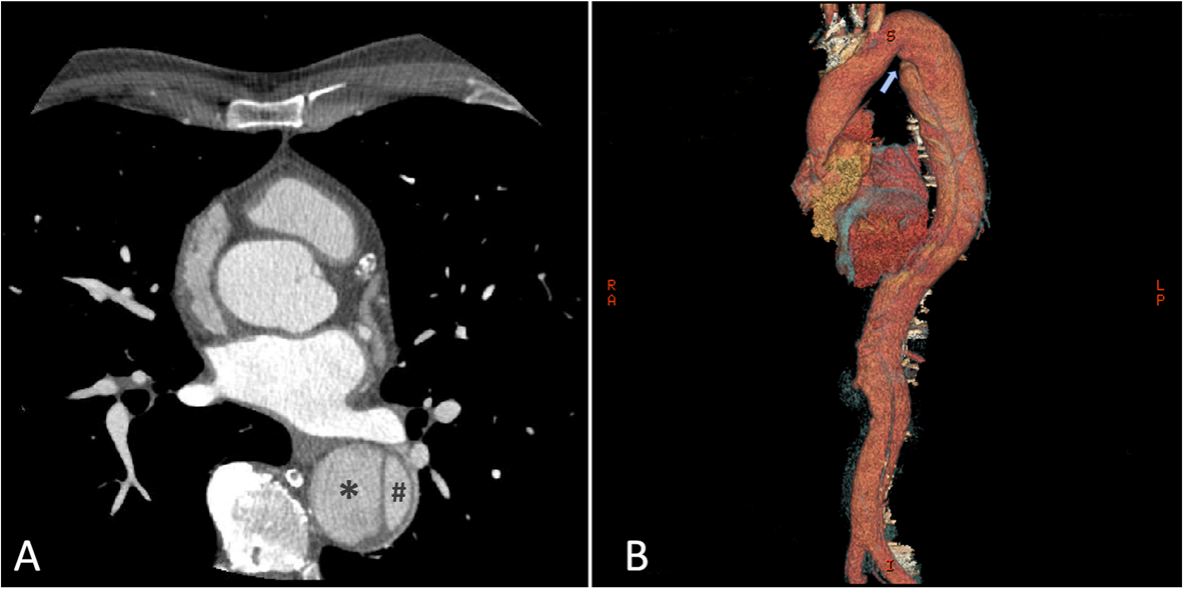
a Axial CTA view showing the TBAD with the false lumen (*asterisk*) clearly prevailing over the true lumen (*hash*). b CTA 3D-scan documenting the extension of the TBAD, originating from the thoracic descending aorta just below the origin of the left subclavian artery (light blue arrow) to the iliac arteries bilaterally
